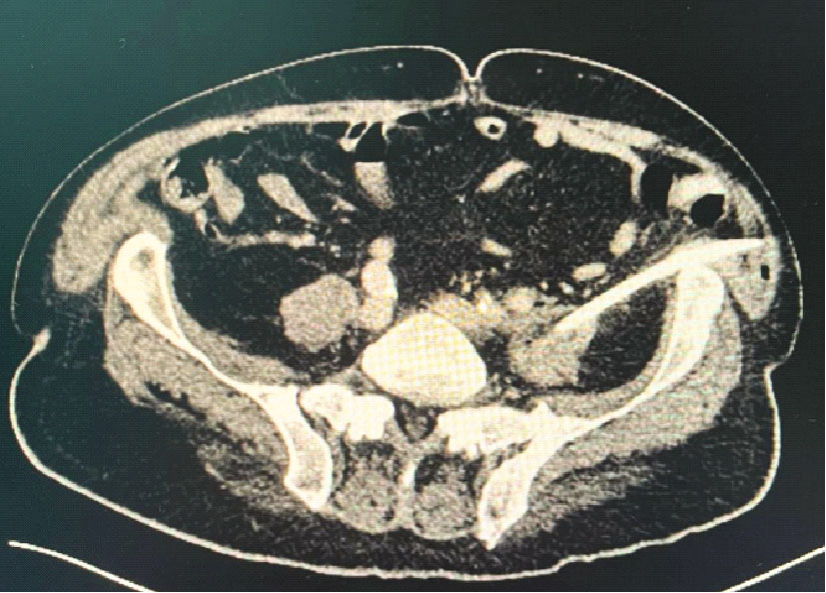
CT-guided retroperitoneal drainage of the psoas' abscess.
